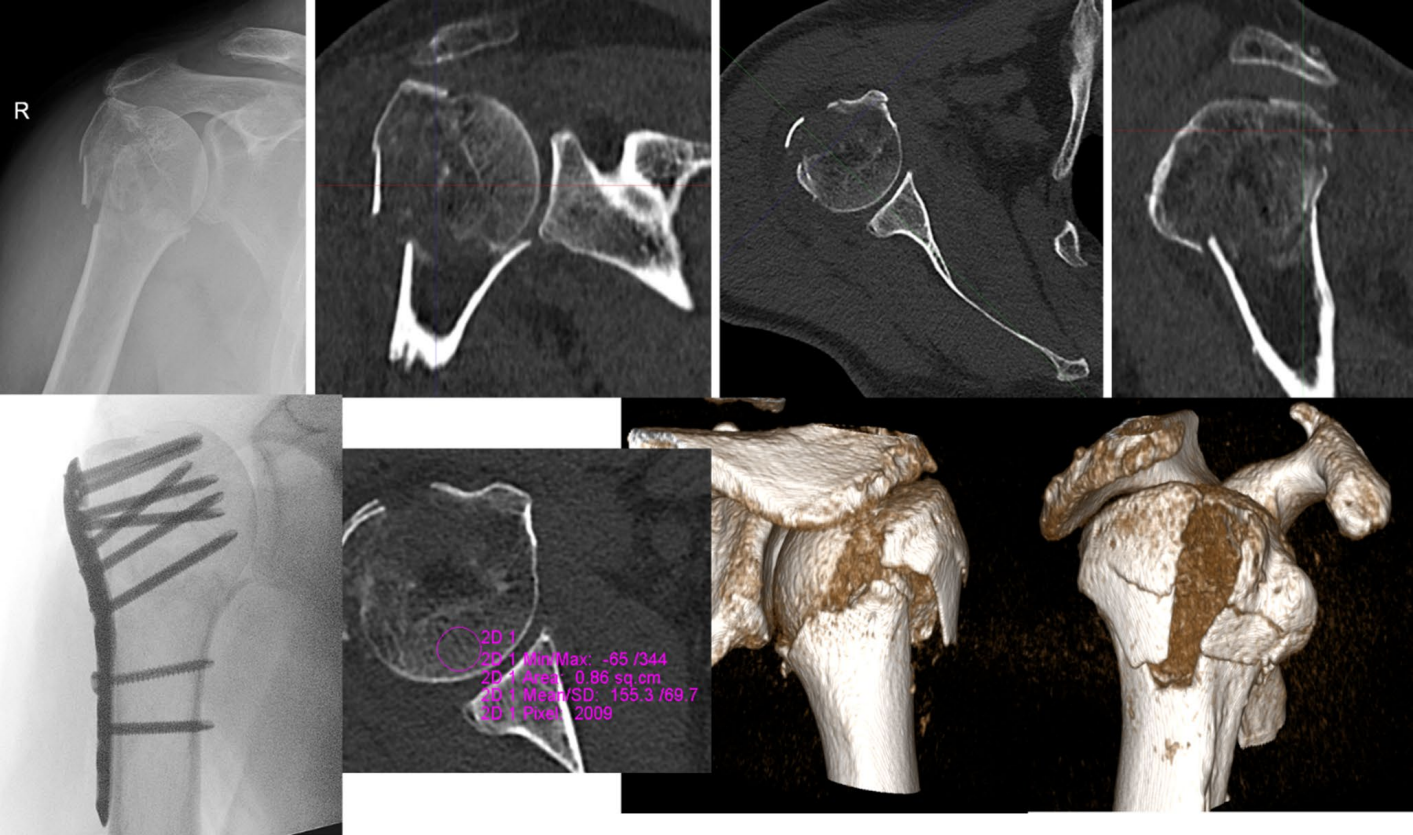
Computed tomography (CT) scan of a four-part proximal humerus fracture in a 66-year-old male with good bone quality (Hounsfield units [HU] > 100), managed with osteosynthesis [40]
